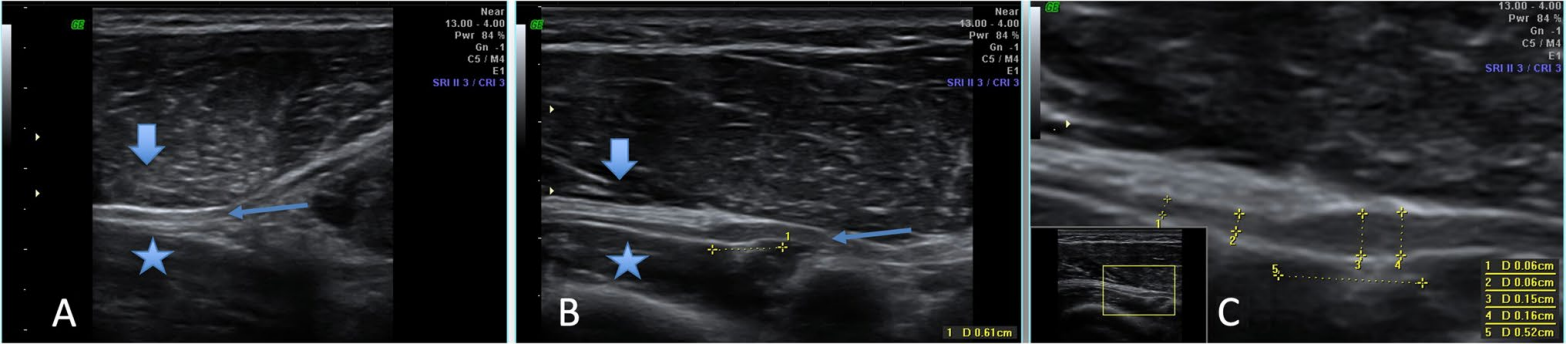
Presents ultrasonographic image of posterior interosseus nerve (PIN). a Normal nerve with regular margins and no deformation. Star and arrow marks deep and superficial supinator head respectively. Thin arrow points posterior interosseus nerve. b Compression of the nerve by arcade of Frohse with significant broadening of the nerve outline (local oedema formation). Star and arrow marks deep and superficial supinator head respectively. Thin arrow points posterior interosseus nerve. c Zoomed view of the changed nerve where comparison of prestenotic nerve edema in comparison with distal part of normal nerve diameter. This is the way of quantification of the nerve enlargement
